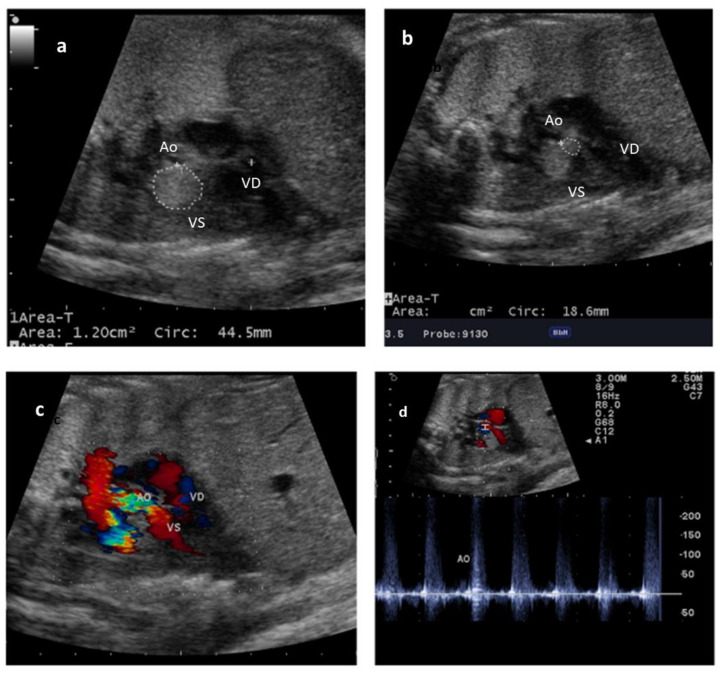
Fetal echocardiography at 32 w.g. A voluminous mass is evident in the left ventricle (VS) close to the inflow (a) and a smaller mass in the outflow toward the aorta (Ao) in the right panel (b). Color Doppler image is seen in panel (c) with a turbulent flow in aorta and pulsed Doppler of aorta in panel (d) with a max gradient of 200 cm/s at pulsed Doppler. VD—right ventricle.
